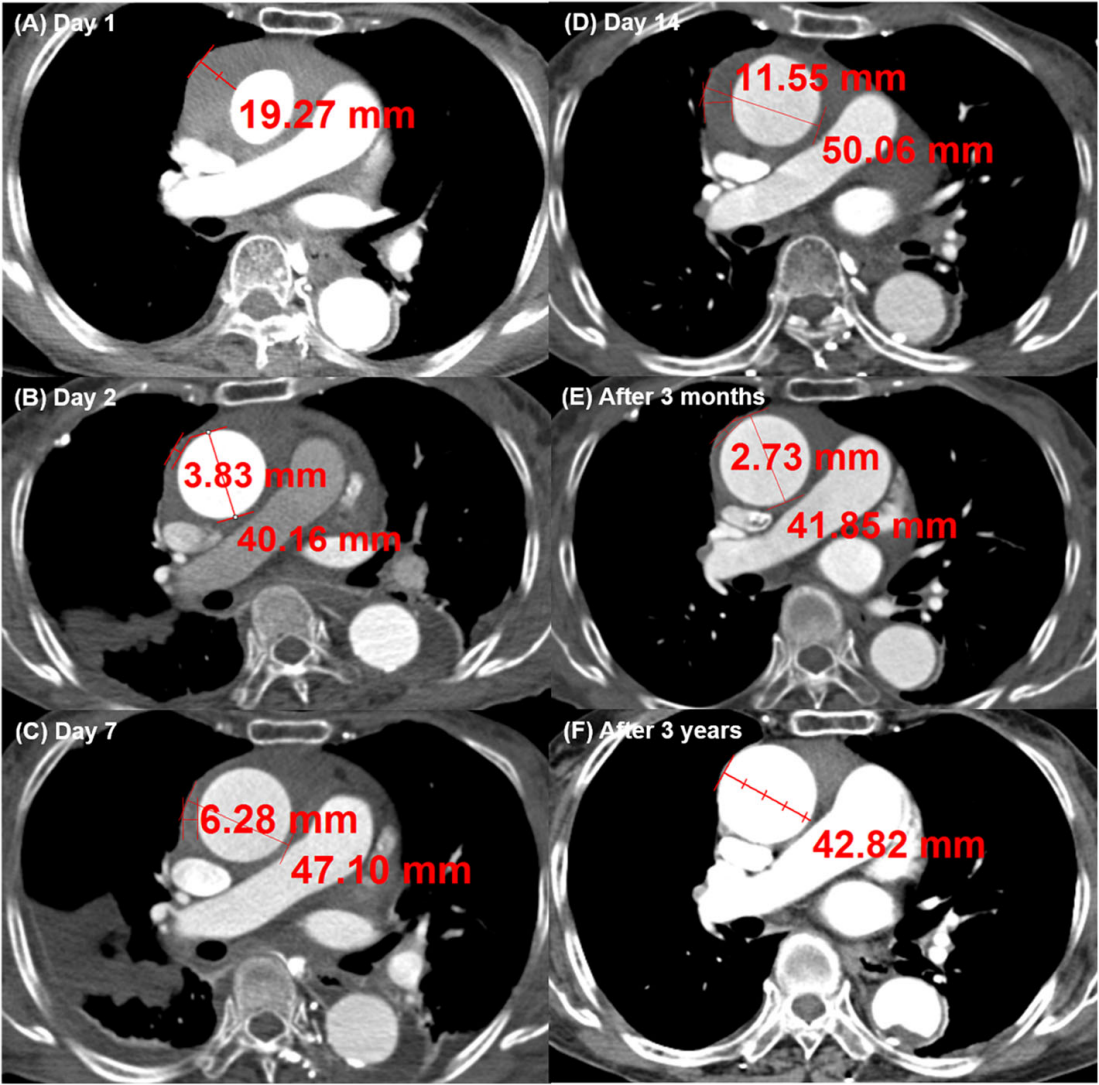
Axial contrast-enhanced computed tomography (CT) showing the change in intramural hematoma (IMH) thickness at the mid-ascending aorta. a-b The day after the occurrence of the catheter-induced aortic dissection, the IMH thickness is noted to decrease from 19 to 4 mm by conservative treatment; c-d However, it gradually increases to 11 mm until the second week. The diameter of the ascending aorta is 50 mm; e-f After 3 months, the IMH thickness has decreased to 2.7 mm. Follow-up CT after 3 years shows complete resolution of the IMH
